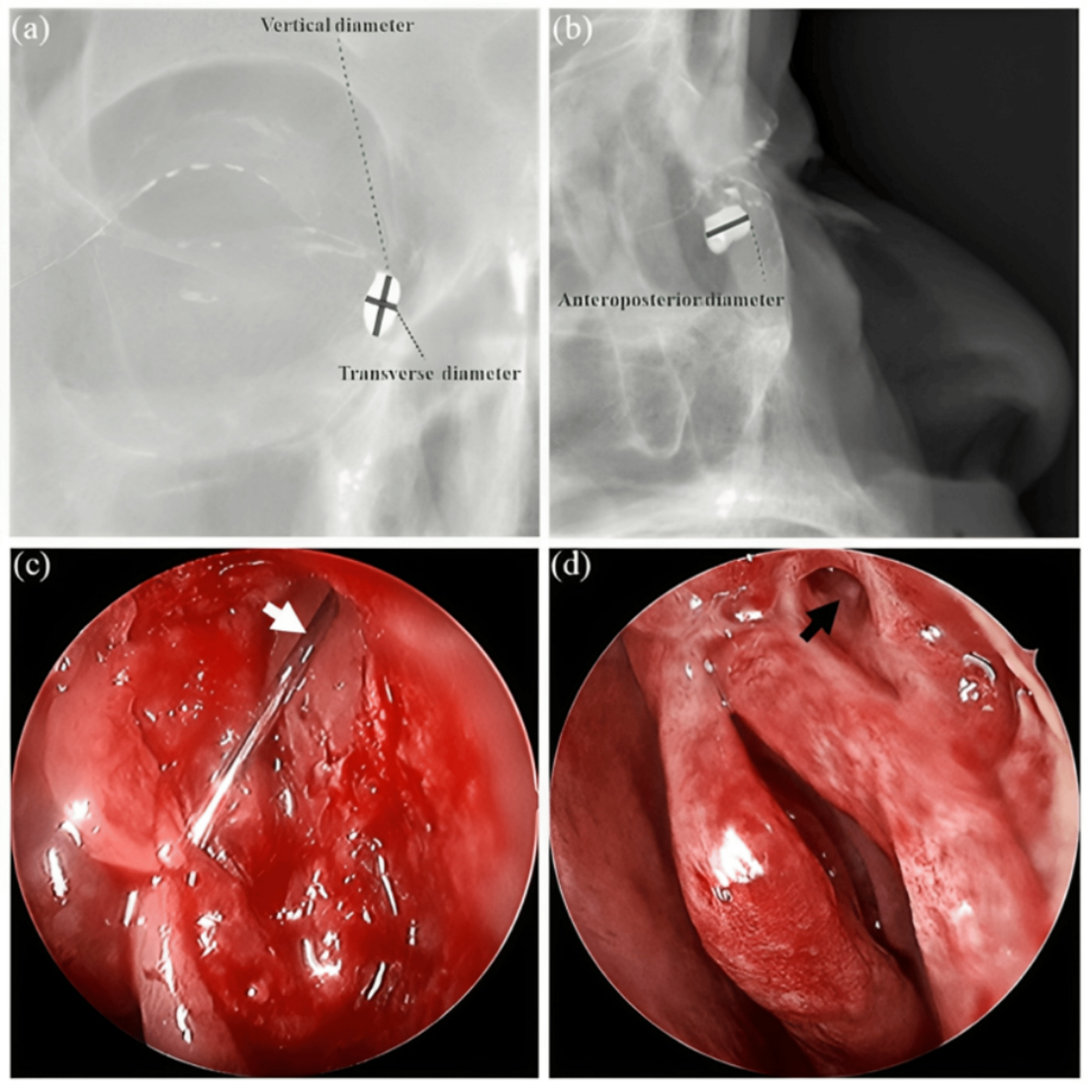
Radiographic and endoscopic findings in nasolacrimal duct obstruction (NLDO). (a) Coronal view of digital dacryocystography showing the vertical and transverse diameters of the lacrimal sac. (b) Sagittal view displaying the anteroposterior diameter. (c) Endoscopic image showing a lacrimal probe passing through the common canaliculus into the sac (white arrow). (d) Postoperative view of the ostium after endoscopic dacryocystorhinostomy (black arrow). Figure from Nie et al., licensed under CC BY-NC-ND 4.0 [17].
